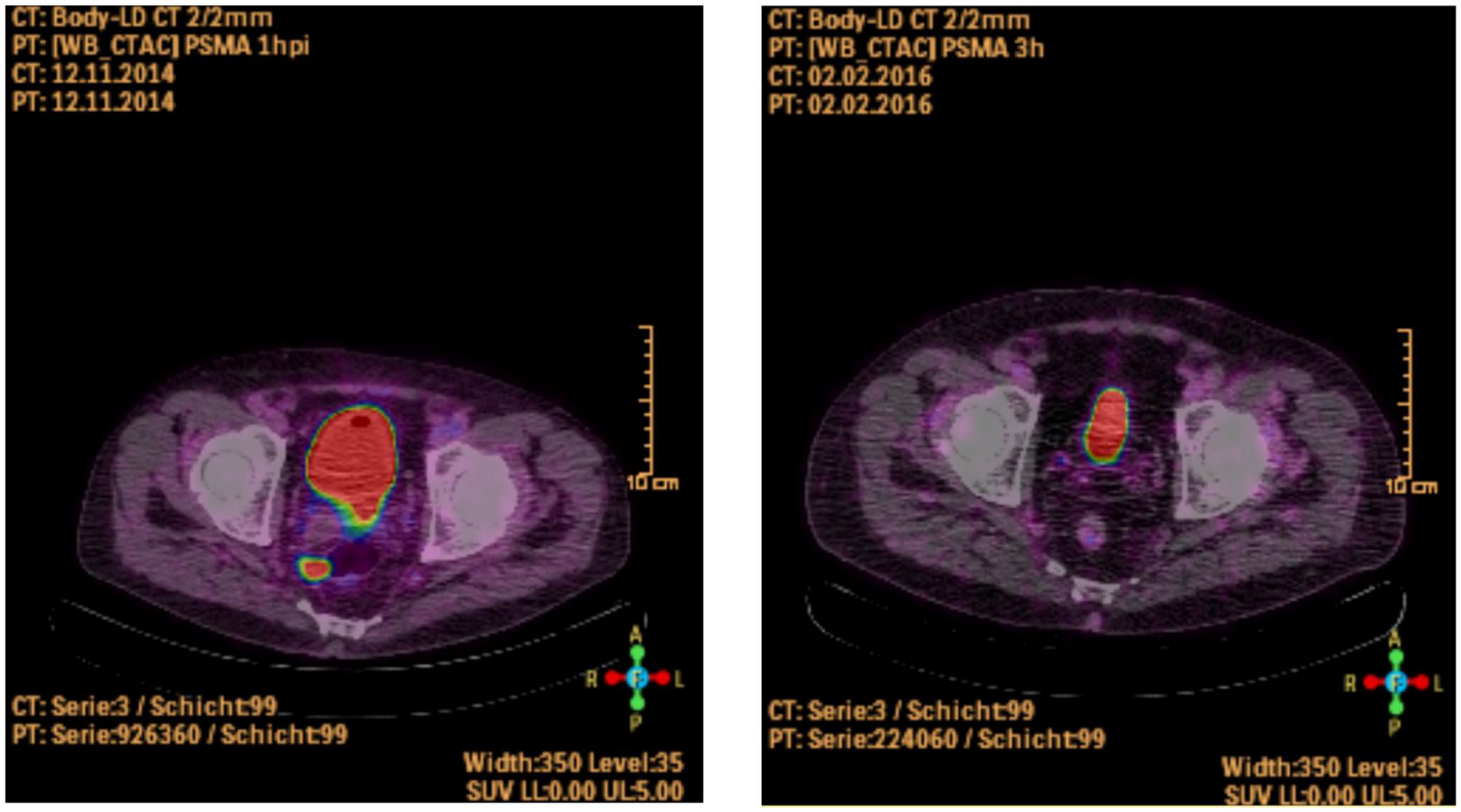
Patient with a singular MLN metastasis in the first ^68^Ga-PSMA-PET/CT (picture on the left side) and showing no more PSMA-uptake following ADT in the second scan after 13 months (picture on the right side, Patient ID 1).
